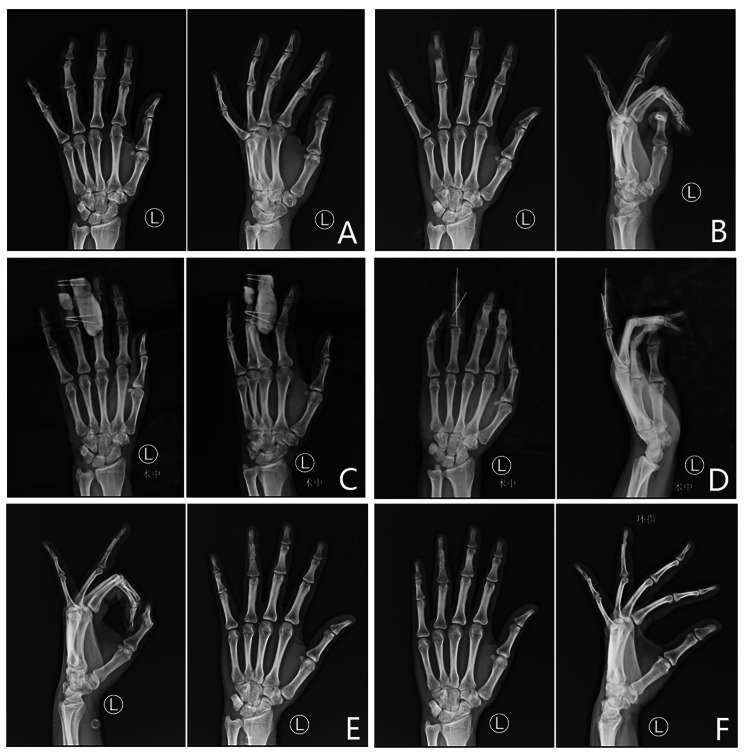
The left ring finger of a 29-year female patient was injured by a bar cutting machine, causing open injury of the distal interphalangeal joint and tendon, nerve, vessel, and soft tissue defects. A, Preoperative X-ray. B, Subsequent posttraumatic osteomyelitis. C, After the sinus and dead bone were removed, the defect was filled with antibiotic PMMA cement fabricated into a cylindrical shape similar to the shape of the phalanx. External fixation was achieved with four transverse and parallel Kirschner wires and additional cement to connect the Kirschner wires. D, At 8 weeks postoperatively, the cement spacer was removed, and the bone graft was carved into a 2.0 cm ⋅ 1.0 cm ⋅ 1.0 cm cylindrical shape similar to the shape of the phalanx. Two Kirschner wires were used to fix the fracture. Distal interphalangeal joint fusion was performed. E, At four weeks postoperatively, callus formation was observed. F, At 10 weeks postoperatively, bone graft union was observed without additional infection
